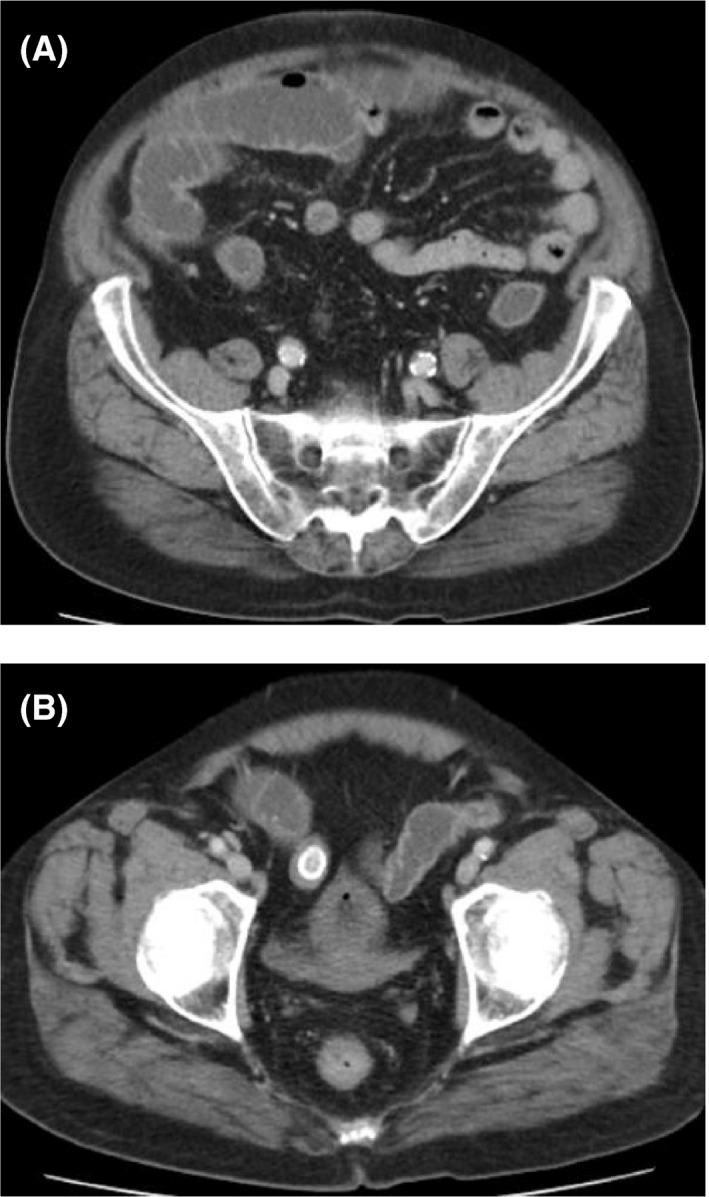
A and B, CT revealed the presence of (A) predominantly dilated proximal loops with the transition point in the terminal ileum at the right iliac fossa; (B) an intraperitoneal large 3 * 4 cm calcified stone at the upper right‐side of the Douglas pouch at the entrance of the minor pelvis
